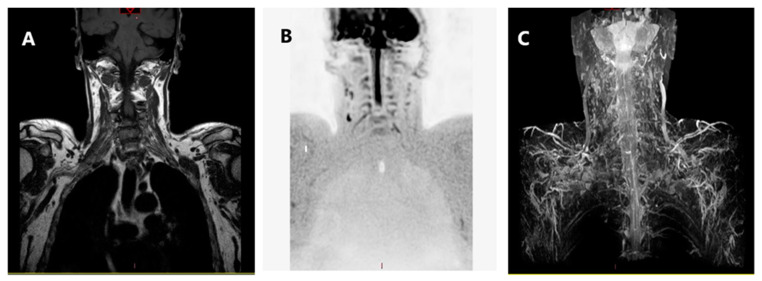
MRI sequences: (A) turbo spin-echo (TSE) T1 cor, (B) DWIBS, and (C) 3D STIR mip.
